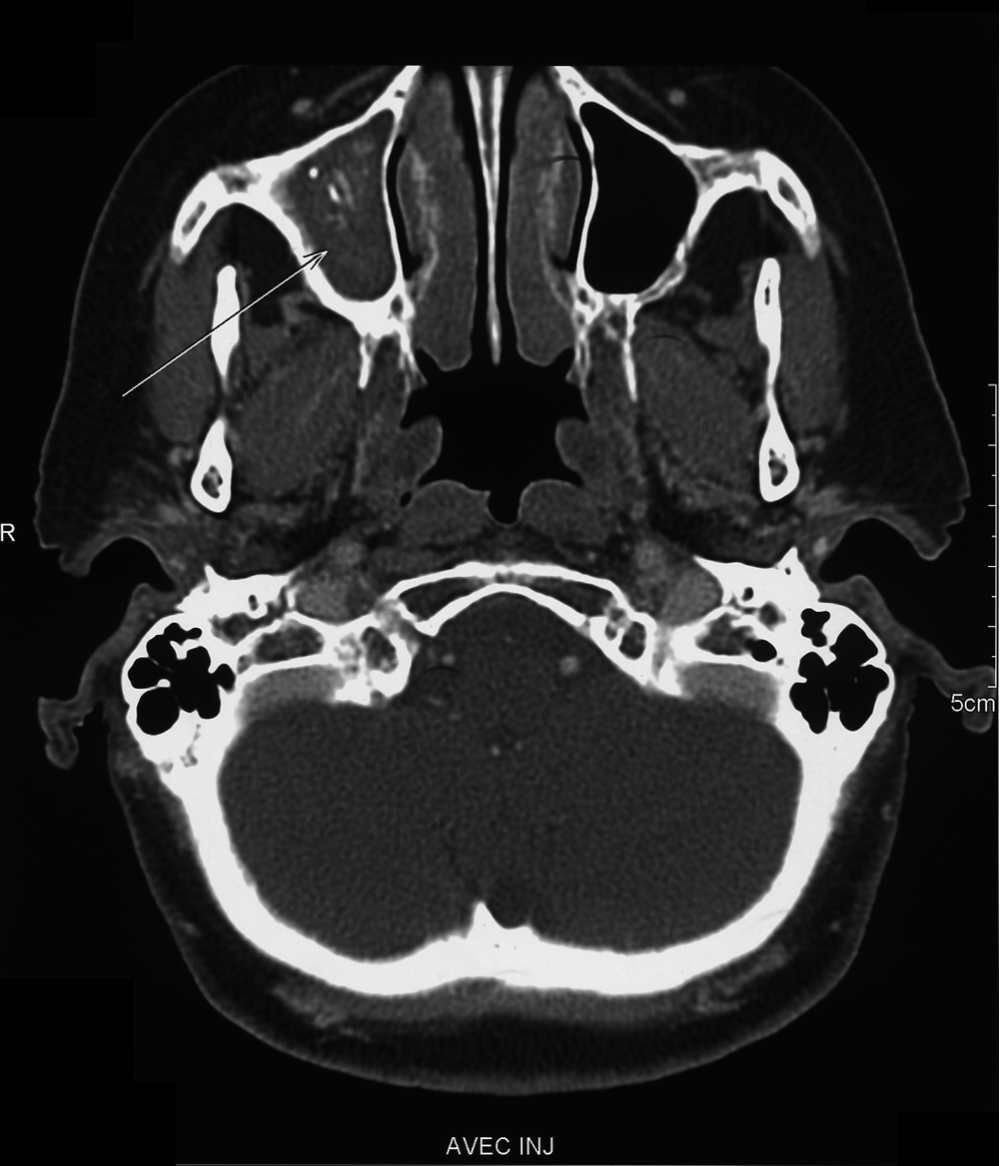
Aspergilloma visible as a soft tissue mass. Computed tomography (coronal view) of the maxillary sinus in Patient 6 before the first surgical procedure. Note the mass containing hyperdense foci that are highly suggestive of aspergilloma (arrow).
